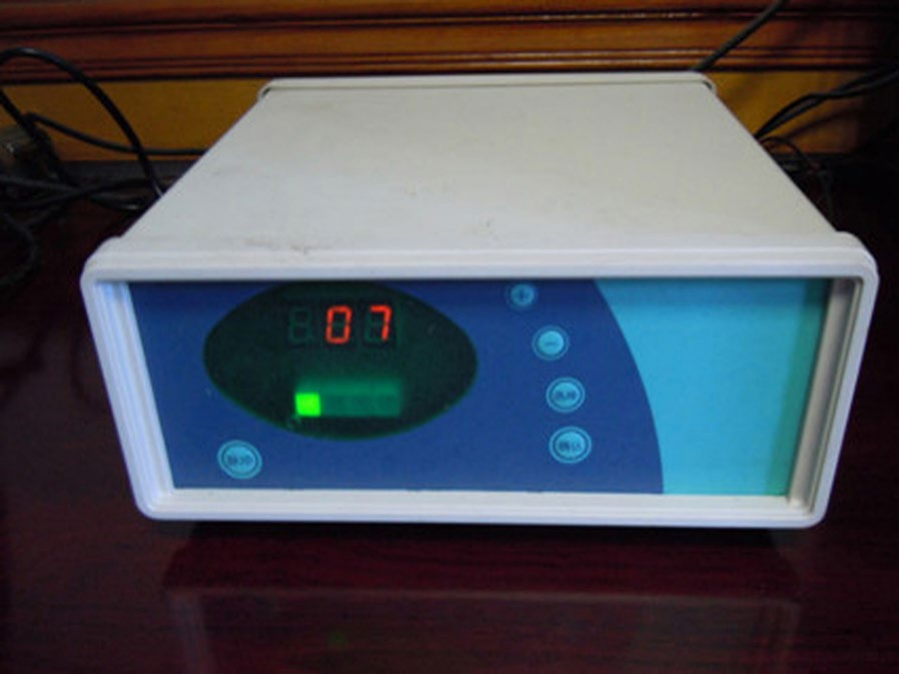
The DZC low-frequency focused ultrasound instrument
